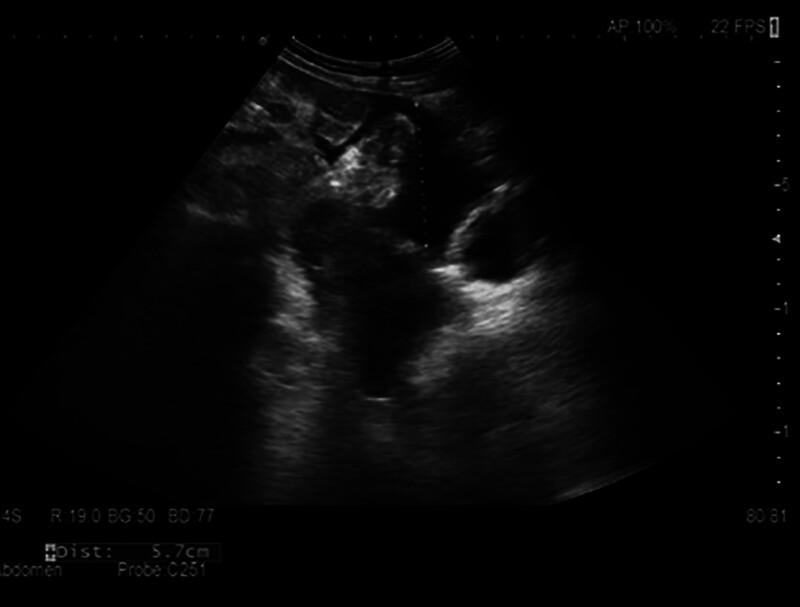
Follow-up ultrasound showing reduction in ascites with maximum depth of approximately 5.7 cm.
